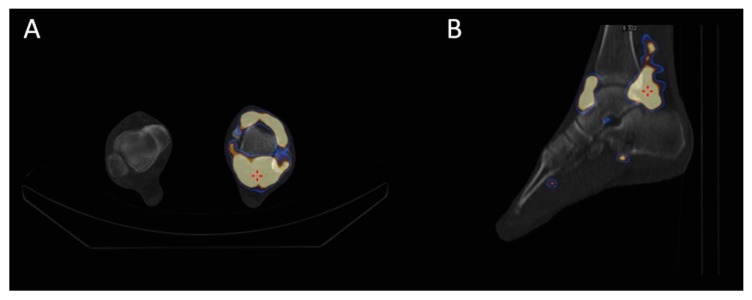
Imaging of the ankle joint performed immediately after intraarticular application of ^169^Er. Fused transaxial single-photon emission computed tomography/computed tomography (SPECT/CT) (A) and coronal SPECT/CT (B) of the ankle joint excluded potential extraarticular leakage of ^169^Er. Due to the very low intensity γ-rays of ^169^Er, imaging was performed with co-administration of 185 MBq of ^99m^Tc in order to render SPECT/CT imaging feasible.
